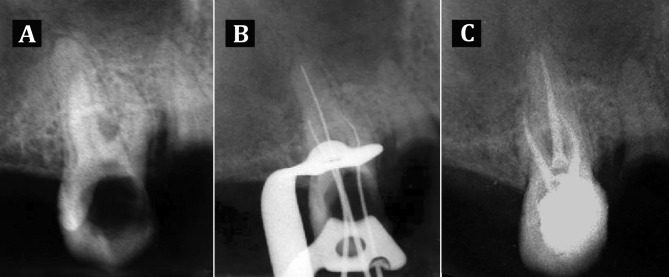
Periapical radiographs of upper right second premolar: A) Carious and radicular lesion; B) working length; C) post-operative radiograph
